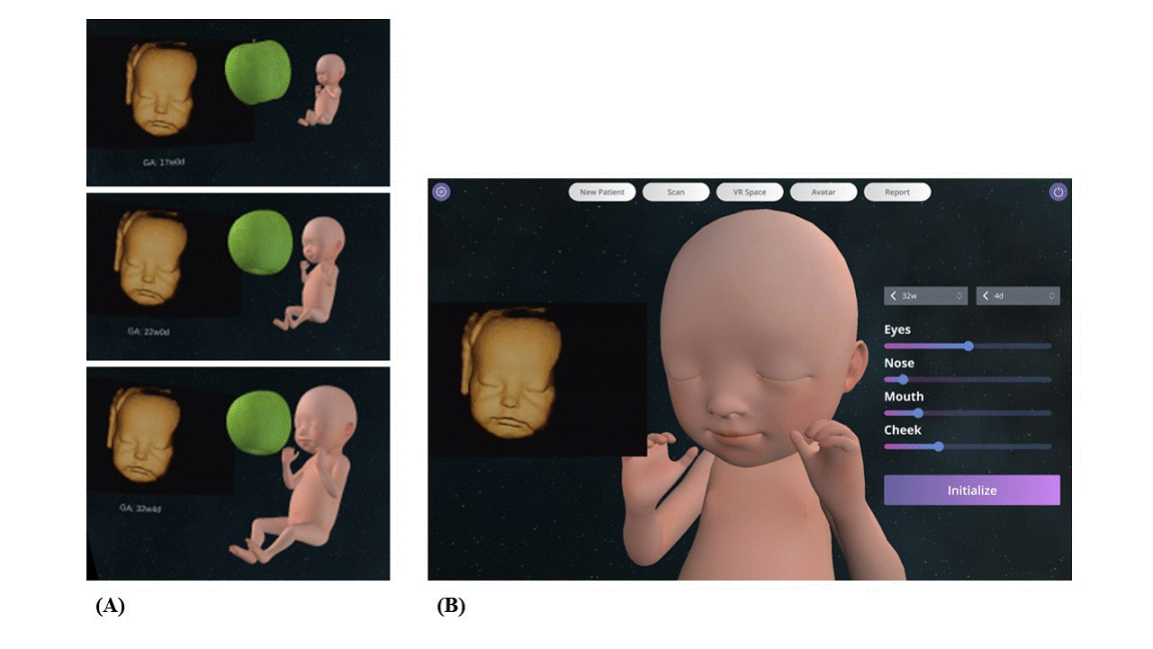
Additional services provided by the prenatal-coaching mobile app for the fetal images produced in virtual reality. (A) Progress in fetal growth at each gestational age in ultrasound images. (B) Interface for users to modify specific structures of the fetal face, such as the eyes, nose, mouth, and cheeks, in virtual reality.
